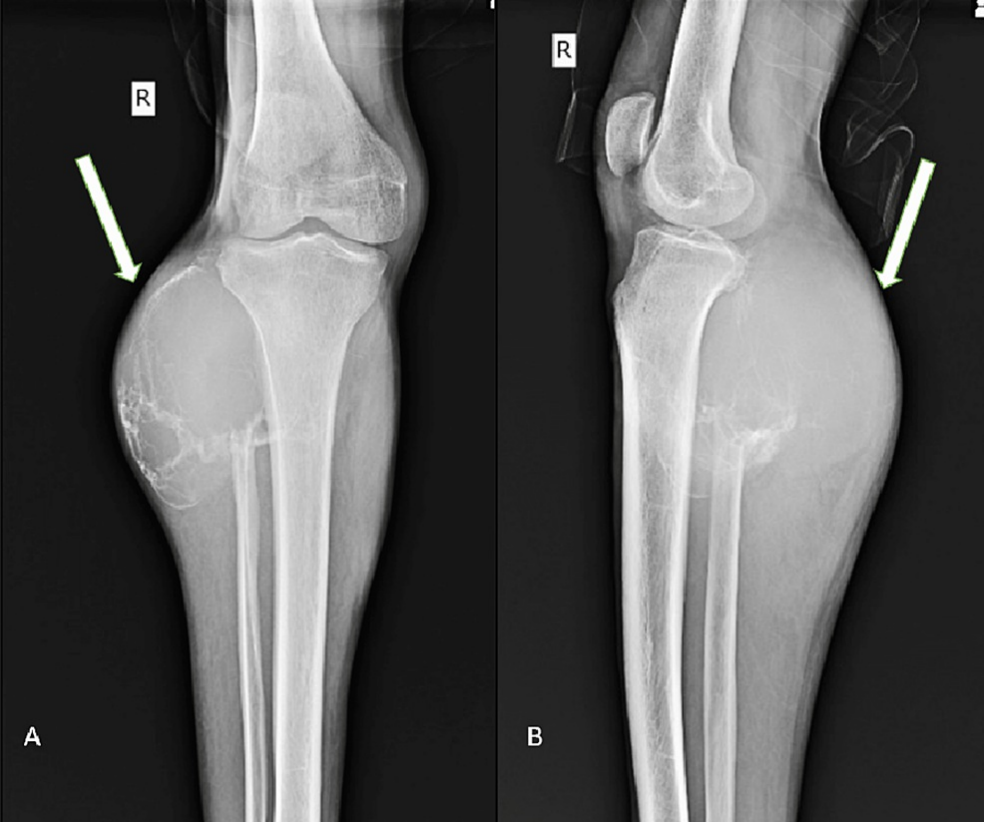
Preoperative X-ray of the tumor. (A) Anteroposterior view of the knee. (B) Lateral view of the right knee.
